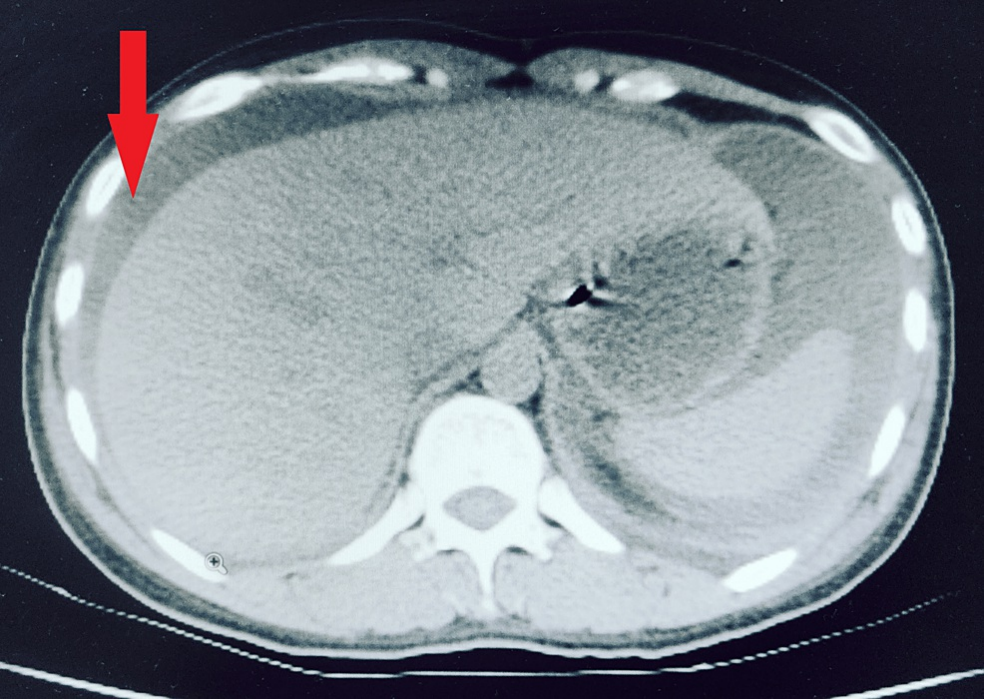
CT image showing ascites marked by arrow
